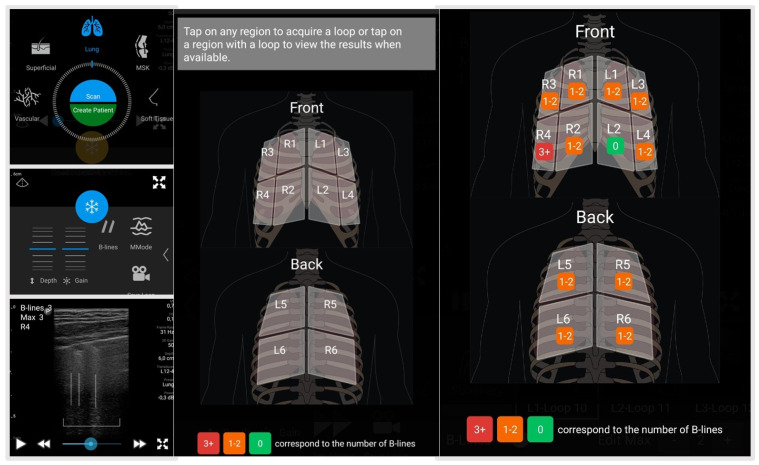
Lung ultrasound scan protocol and mode selection in this study (from [26]).
